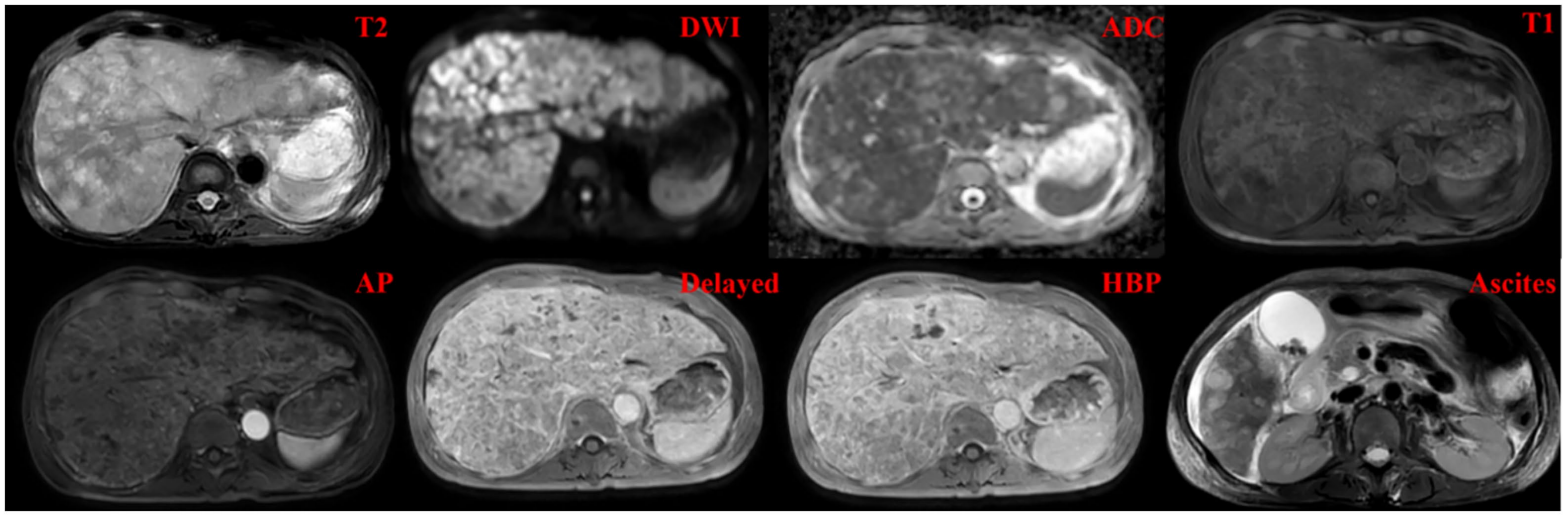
MRI of the liver: T2WI, DWI, apparent diffusion coefficient (ADC), T1WI, contrast-enhanced arterial phase, delayed, and hepatobiliary phases, and ascites after treatment.
